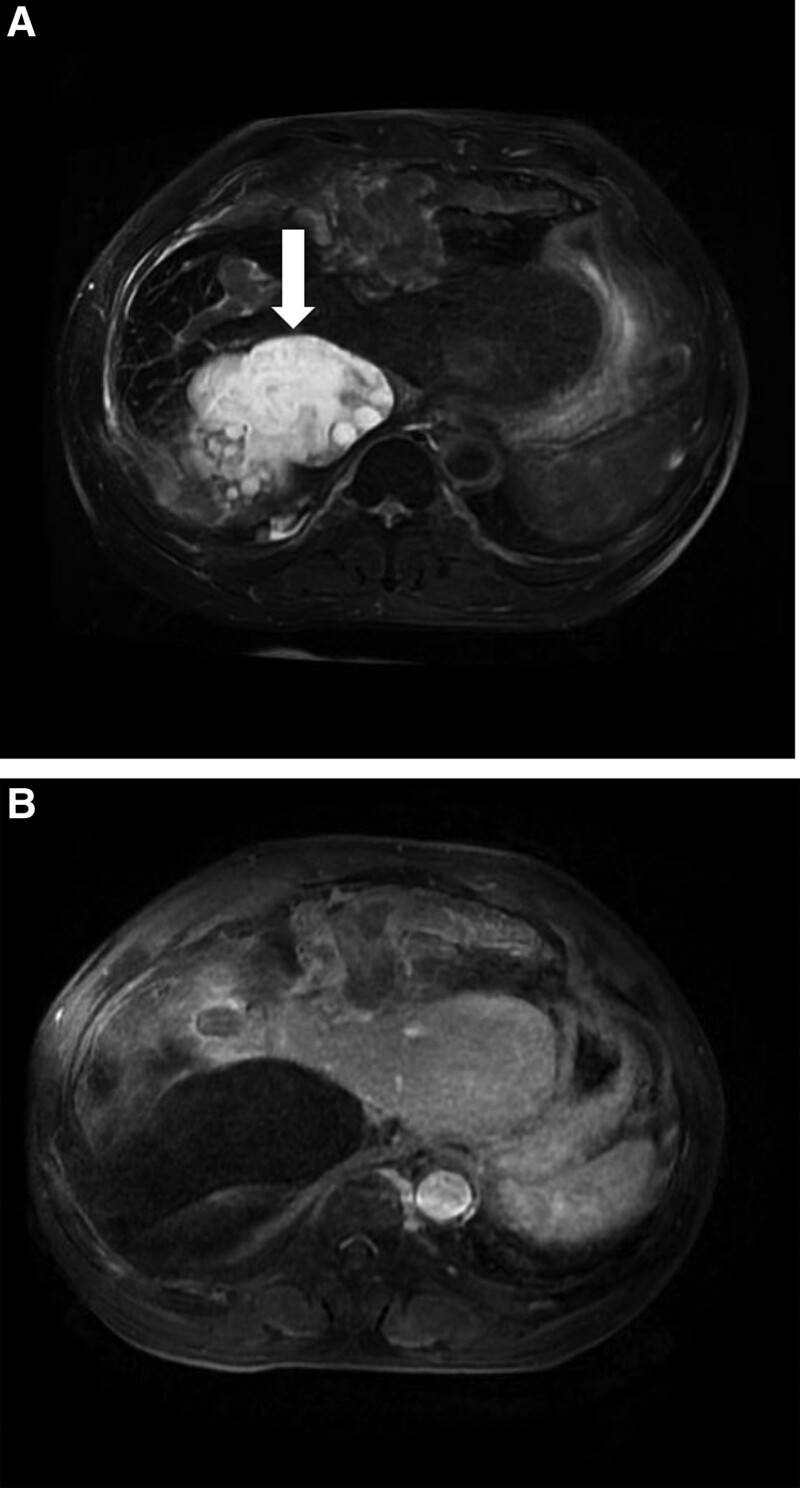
(A and B) Preoperative abdominal magnetic resonance imaging (MRI) images (the white arrow direct the large cystic hydatide of the right lobe of the liver).
